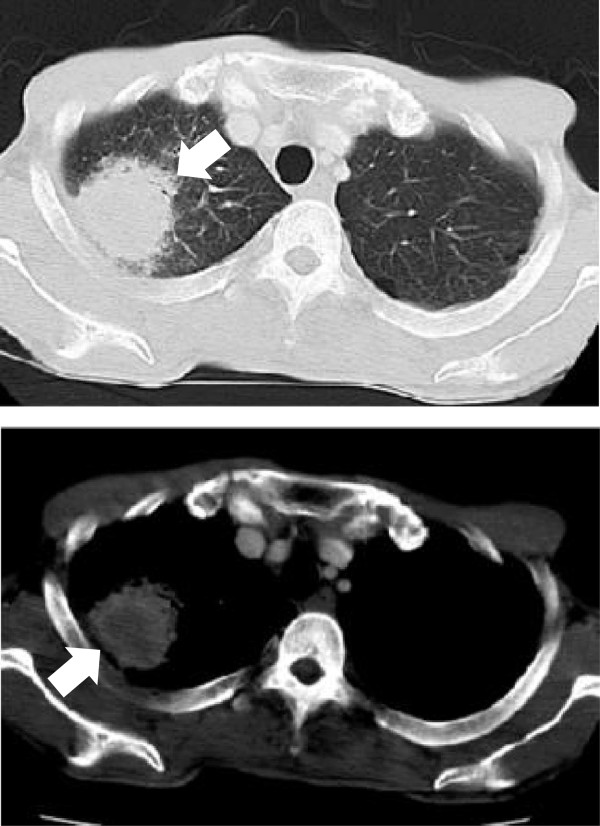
Chest CT scan on admission. Arrow: tumor.
